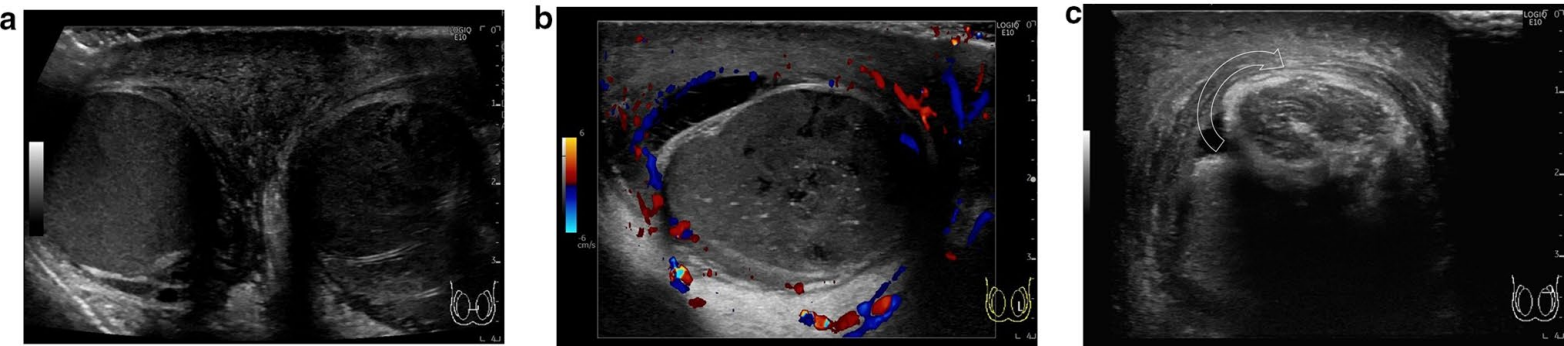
Ultrasonographic images of a 13-year-old boy with testicular torsion. a The left testis is enlarged and shows heterogenous echogenicity. b Vascular flow is absent within the affected testis. c The spermatic cord is twisted in the outer direction. This direction is considered atypical. Surgical exploration was performed, and the outer direction of the spermatic cord twist was confirmed
